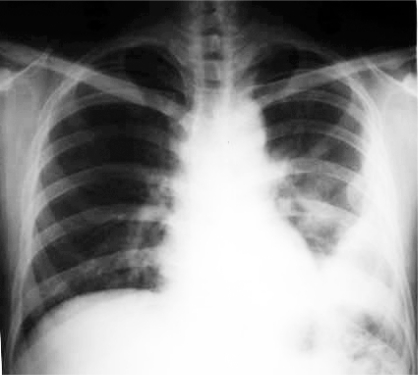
X-ray chest showing left pleural based mass.
